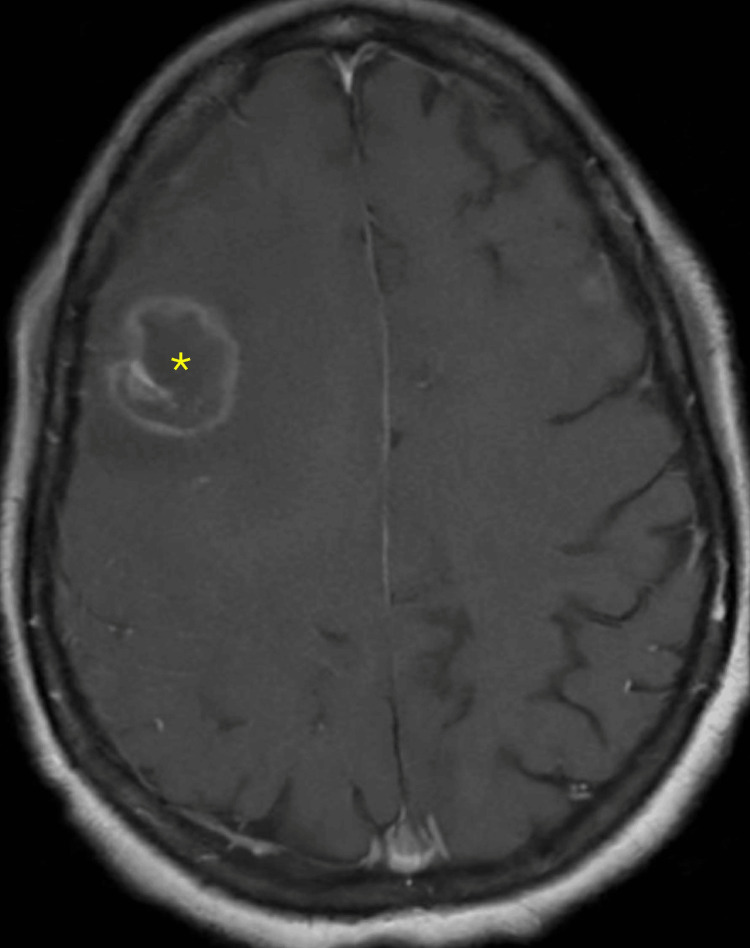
Initial brain MRI with and without contrast A ring-enhancing lesion with edema and mass effect is indicated by the yellow asterisk.
